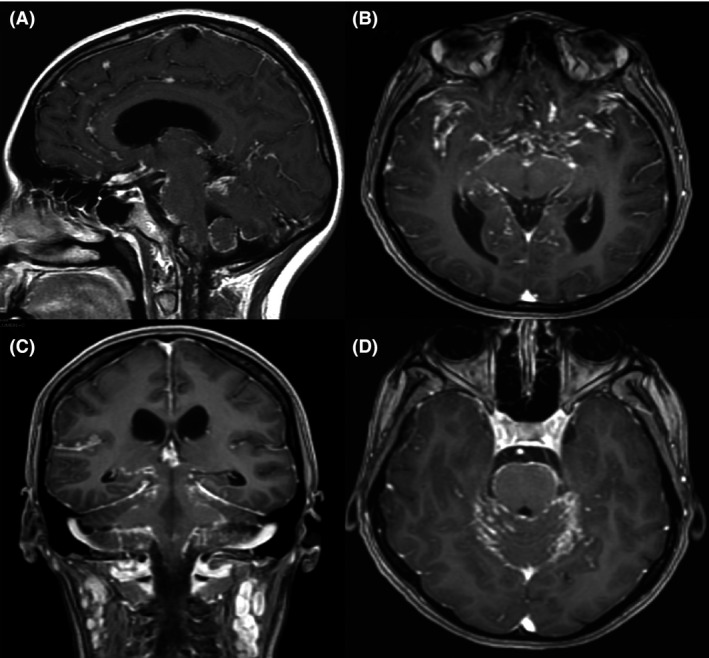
Brain Magnetic Resonance Imaging. T1‐weighted images after administration of gadolinium in the sagittal (A), coronal (C), and axial (B and D) planes. Generalized leptomeningeal reinforcement of basal predominance and multinodular appearance; hydrocephalus is also observed.
